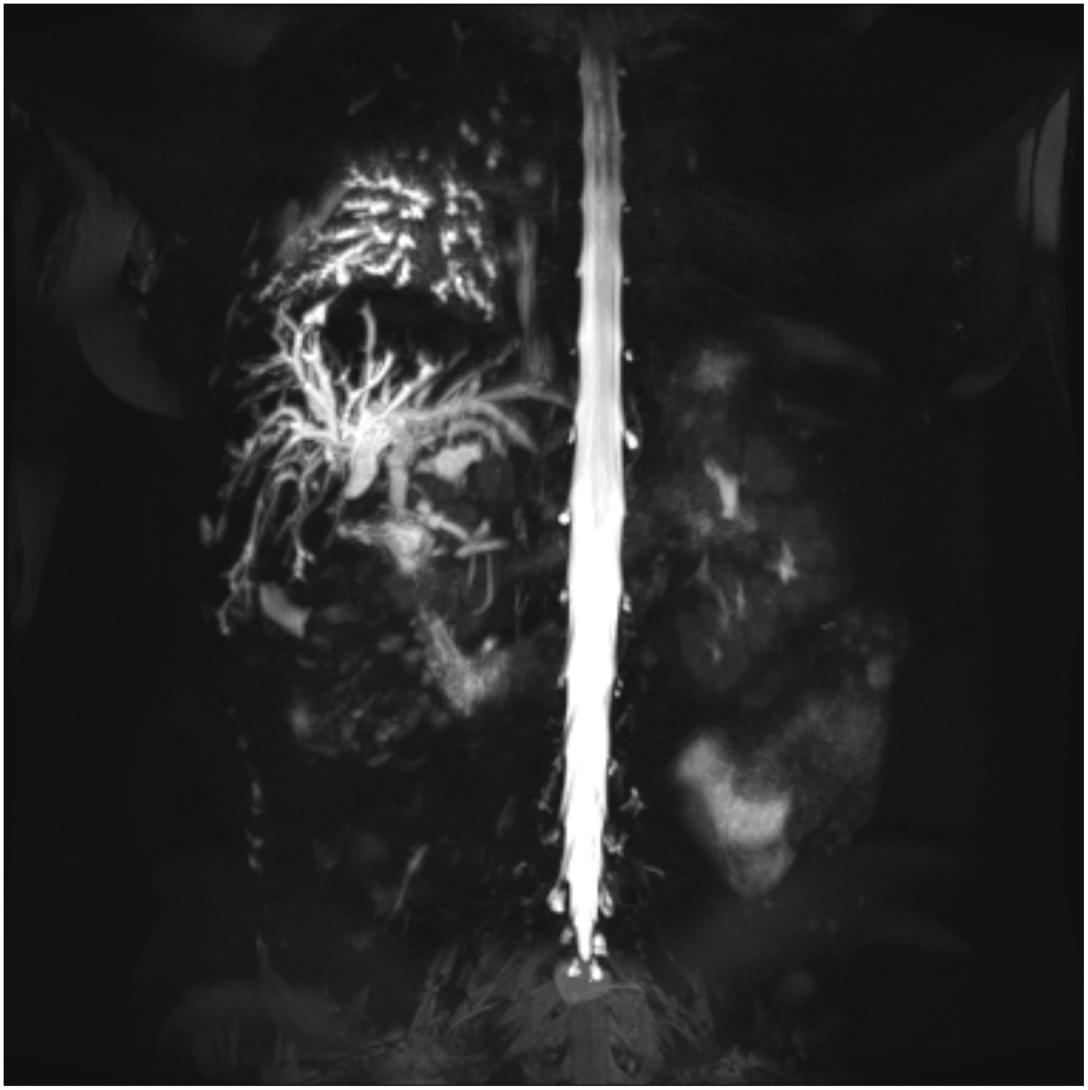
Cholangiography—MRI showing the fistulous path.
